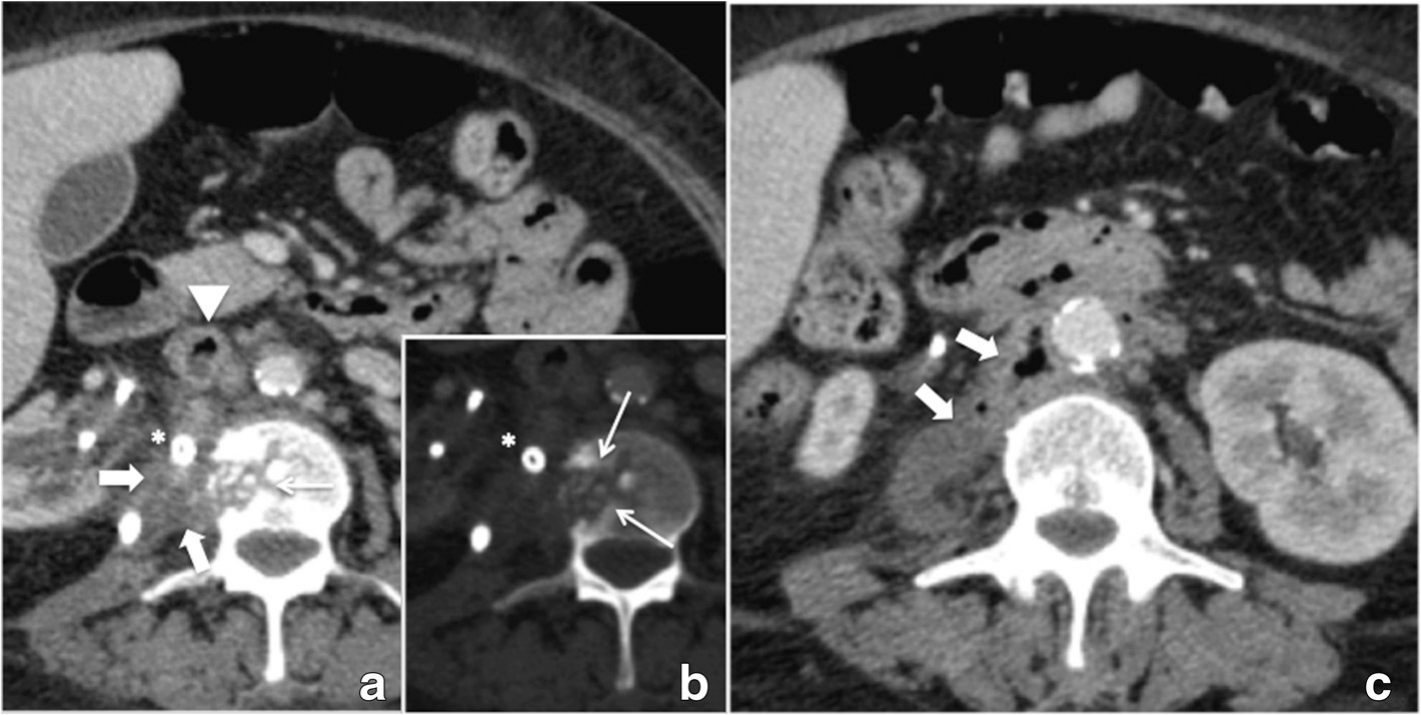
consecutive follow-up CT scan showing constant size of the abscess (thick white arrow) (a, c), progressive osteolytic lesion (small white arrow) (b), and infectious thrombus in the inferior vena cava (white arrowhead)
